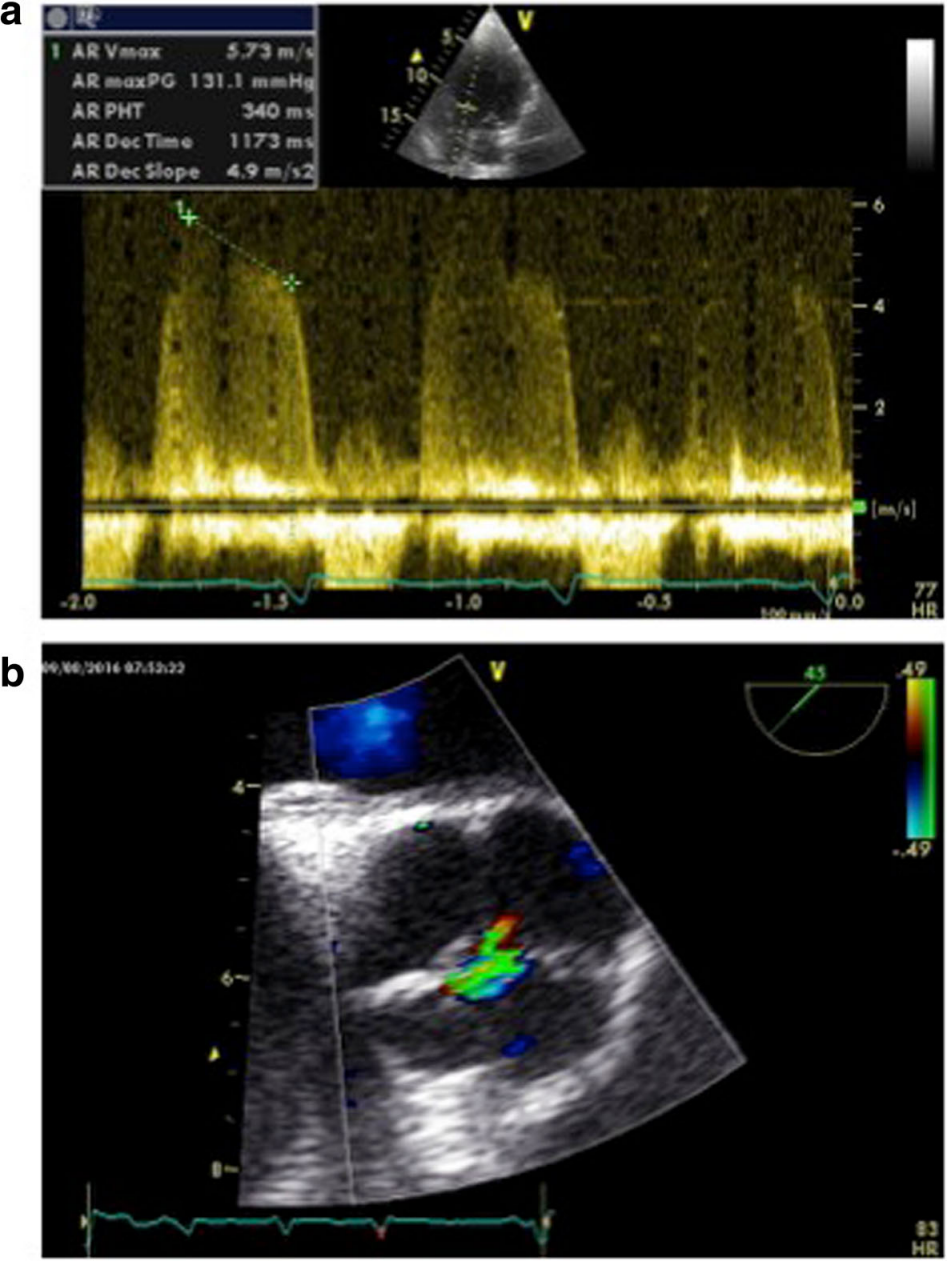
Echocardiography: severe aortic valve regurgitation (a) CW Doppler (b) Color Doppler
